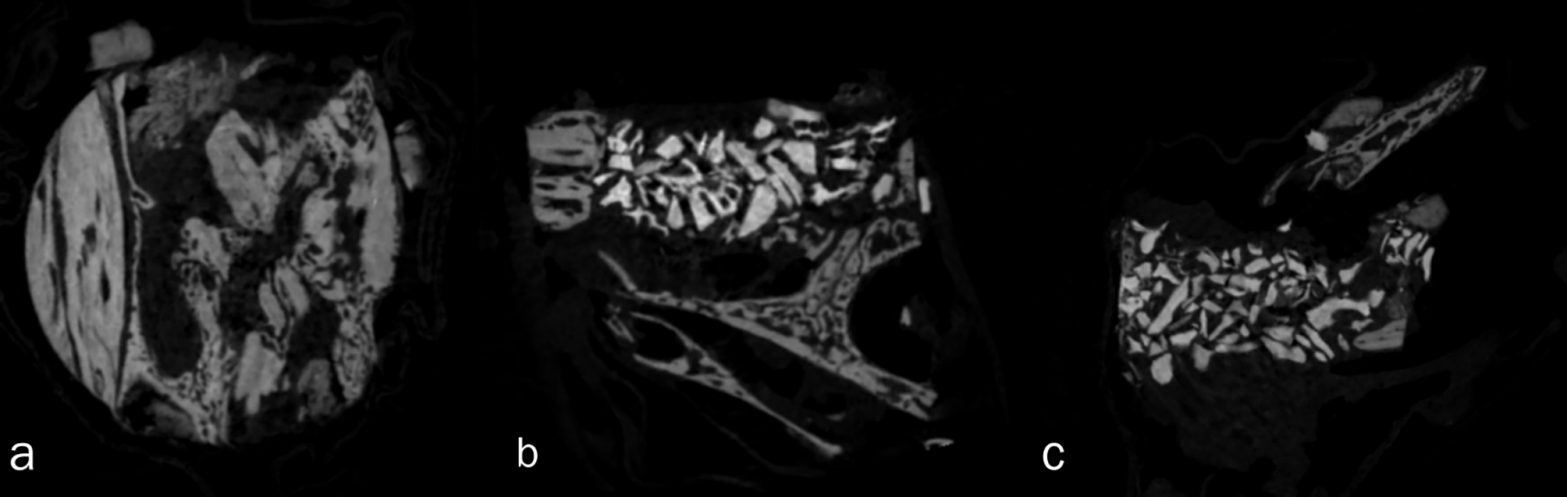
Micro CT images of the graft sites. A) Autologous graft, B) fHAP, C) bHAP
